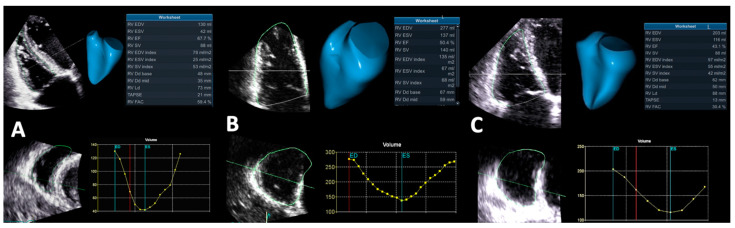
Right ventricular volumes and ejection fraction calculation by three-dimensional echocardiography in (A) a normal subject, (B) a patient with right ventricular dilation and preserved systolic function, and (C) a patient with right ventricular dilation and dysfunction. Abbreviations as in Figure 1.
